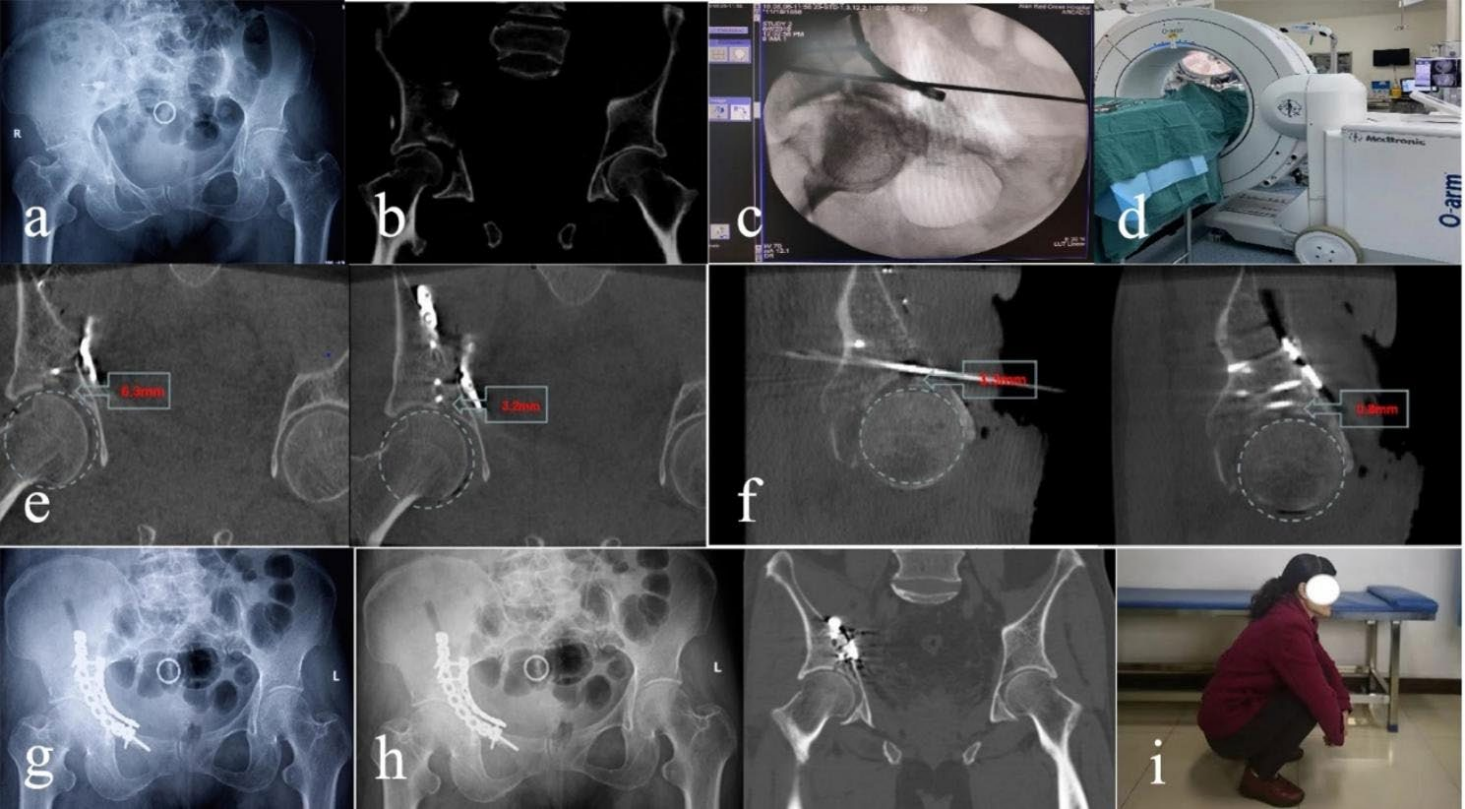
A female patient, aged 64, who presented with anterior column and posterior hemi-transverse fracture from a walking fall injury. (a, b) Preoperative X-ray and CT scan revealed acetabular dome impaction fractures. (c) X-ray showed good consistency of the head and socket after initial reduction and satisfactory reduction of the fracture. (d) Intraoperative O-arm scan was used to assess fracture reduction. (e) The residual gap displacement of the acetabular dome was reduced from 6.3 mm to 3.2 mm in the first and last O-arm scans. (f) The residual step-off displacement of the acetabular dome was reduced from 3.3 mm to 0.8 mm in the first and last O-arm scans. (g) Postoperative X-ray showed satisfactory reduction of the fracture. (h) X-ray and CT scans 11 months after surgery showed union. (i) The affected hip functioned well 11 months after surgery
